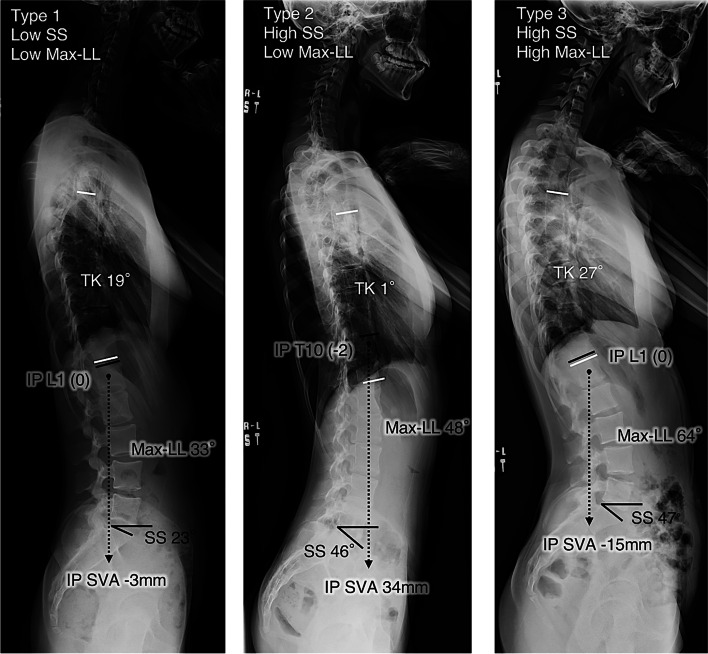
Lateral X-ray films of representative cases. Type 1 is generally manifests with a flat sagittal profile with a smaller SS and Max-LL. Type 2 demonstrates a larger SS, small Max-LL and a significantly small TK. Type 3 shows a large SS and Max-LL
